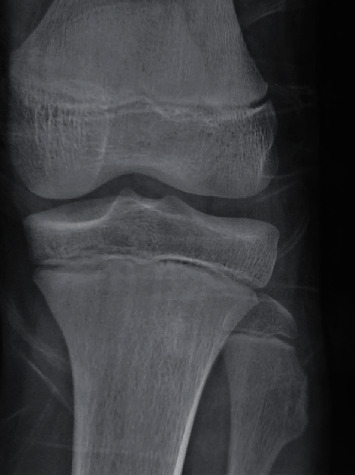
Anteroposterior plain films of the left knee showing an osteophytic protuberance in the neck of the fibula.
